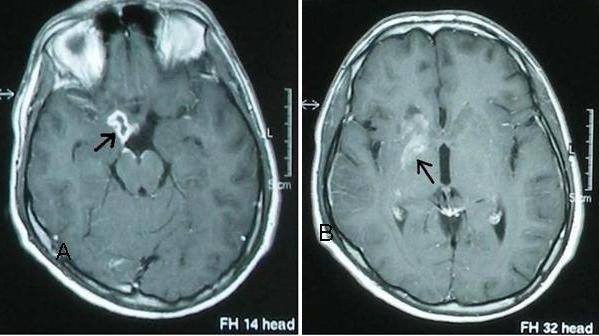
Post contrast T1-weighted MRI shows (A) a granuloma with peripheral enhancement in the genus of the right capsula interna, and (B) an infarct in the basal ganglion due to vasculitis .
